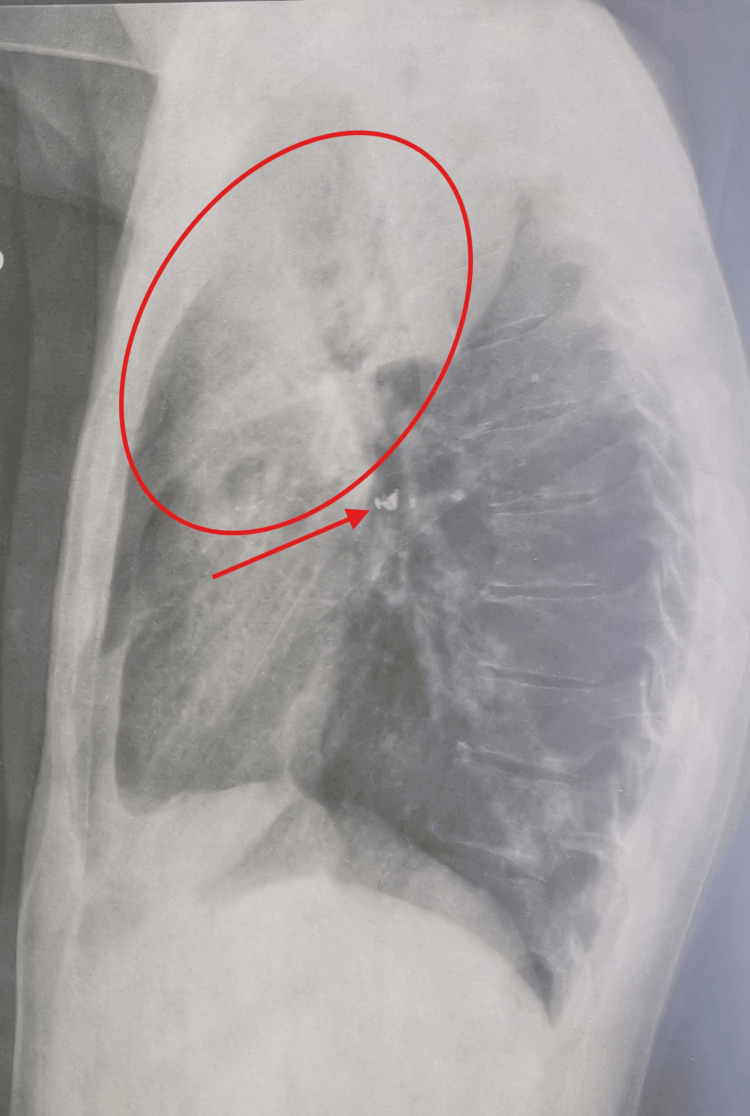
Lateral chest X-ray confirming the presence of a calcified opacity projected over the right upper lobe bronchus (red arrow), associated with right upper lobe consolidation (red circle).
